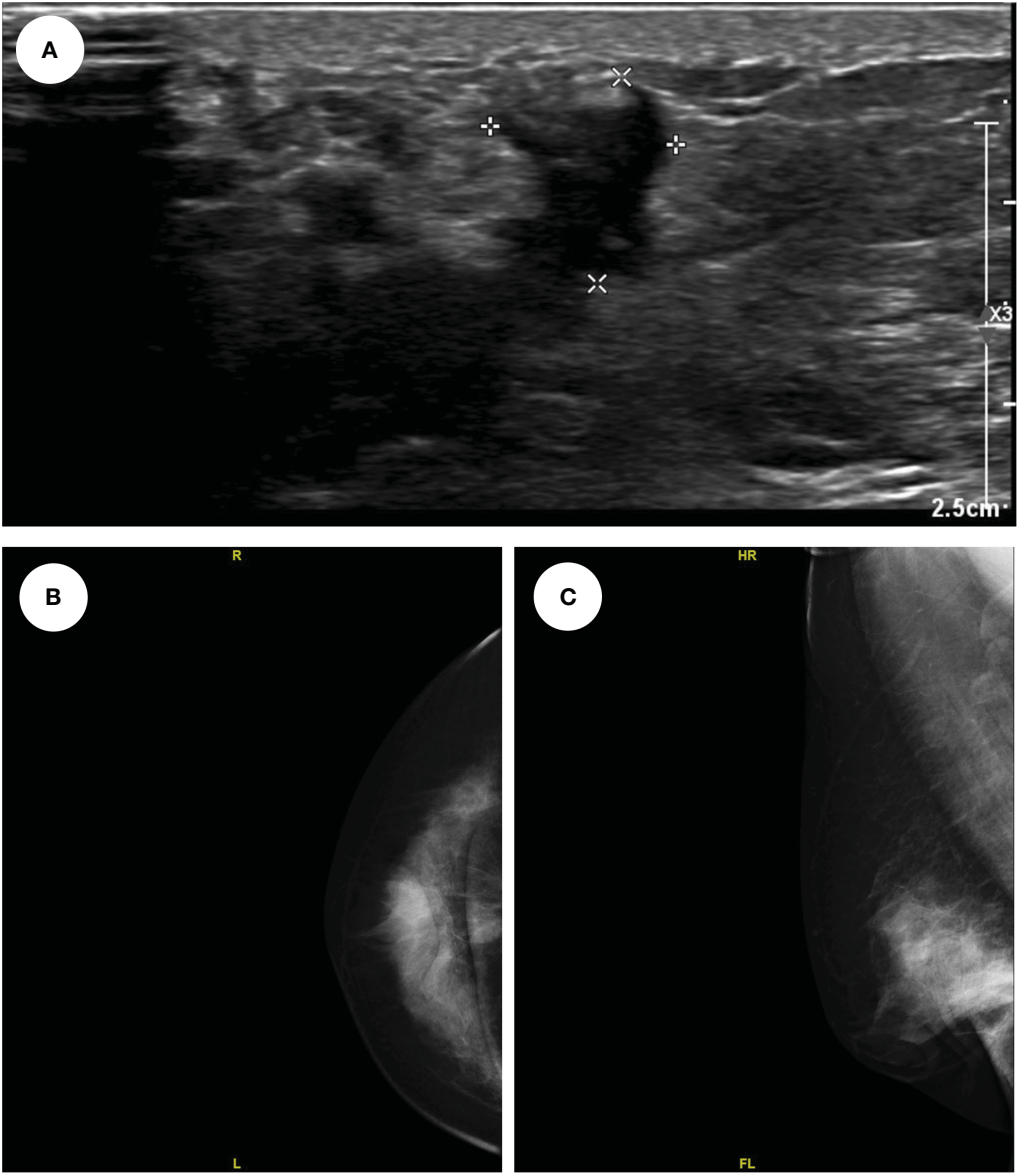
The ultrasound showed a 1.5*0.9*1.0cm hypo echoes irregular mass located in the 5 o’clock position of the right breast (A); The mammogram showed a mass in the inner lower quadrant of the right breast (CC, B); (MLO, C).
